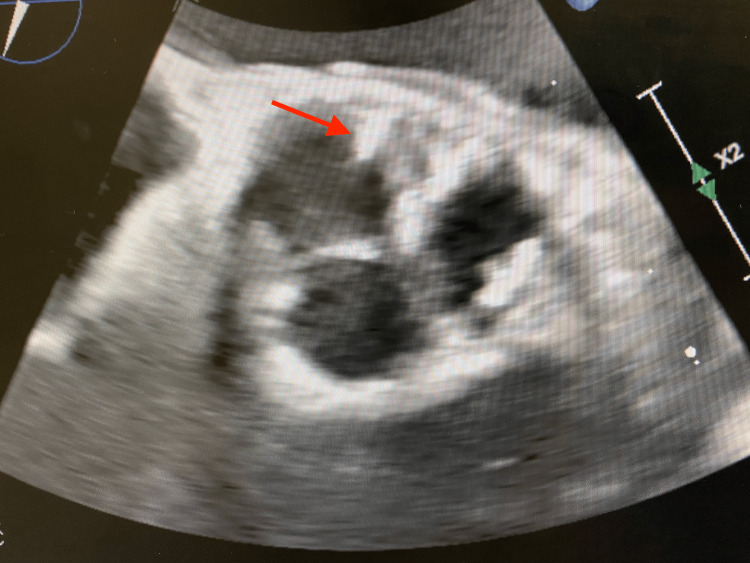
Transesophageal echocardiogram depicts vegetation on the aortic valve (red arrow).
